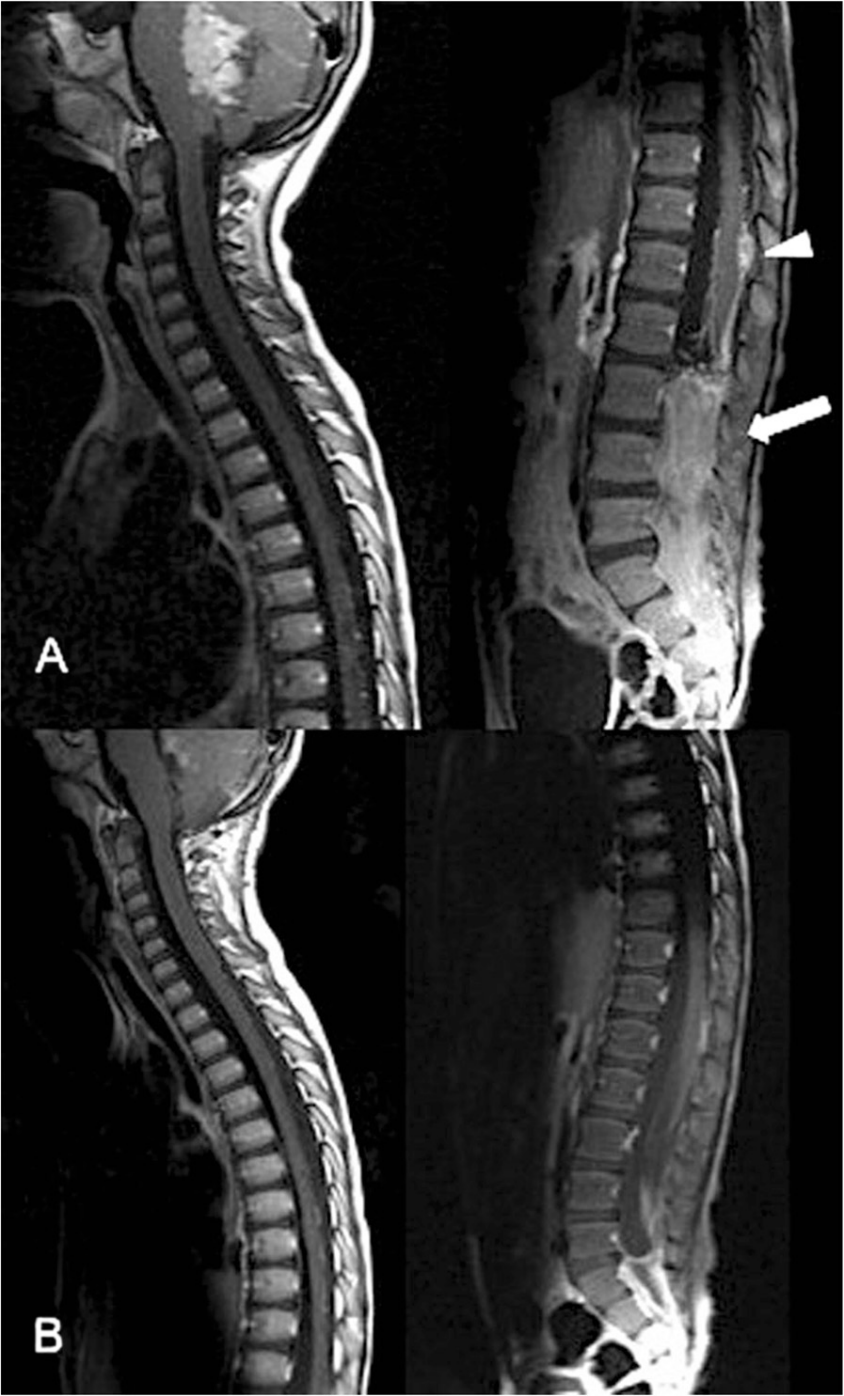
(A) Pre-treatment spinal T1-weighted image with gadolinium demonstrating a nodular extra-medullary enhancement (arrowhead) and spinal block in the lumbar region (arrow). (B) Post-treatment one-year follow-up spinal magnetic resonance imaging revealed regression of the disease.
